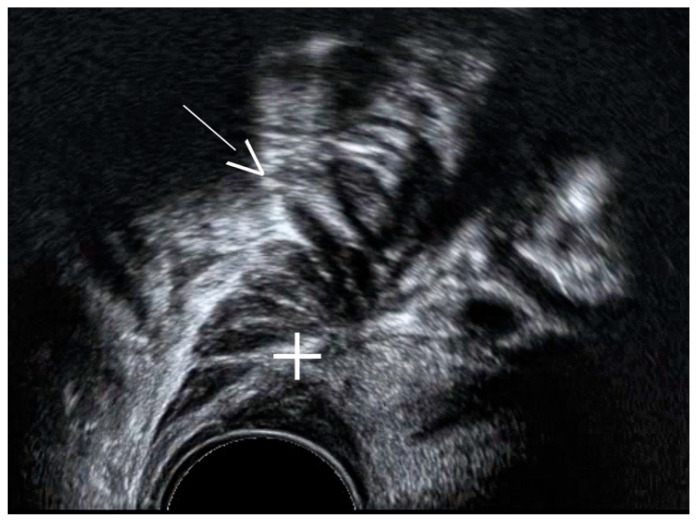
Ultrasound image showing a DIE nodule (+) with prominent spikes towards the bowel lumen (white arrow, “Indian headdress sign”).
